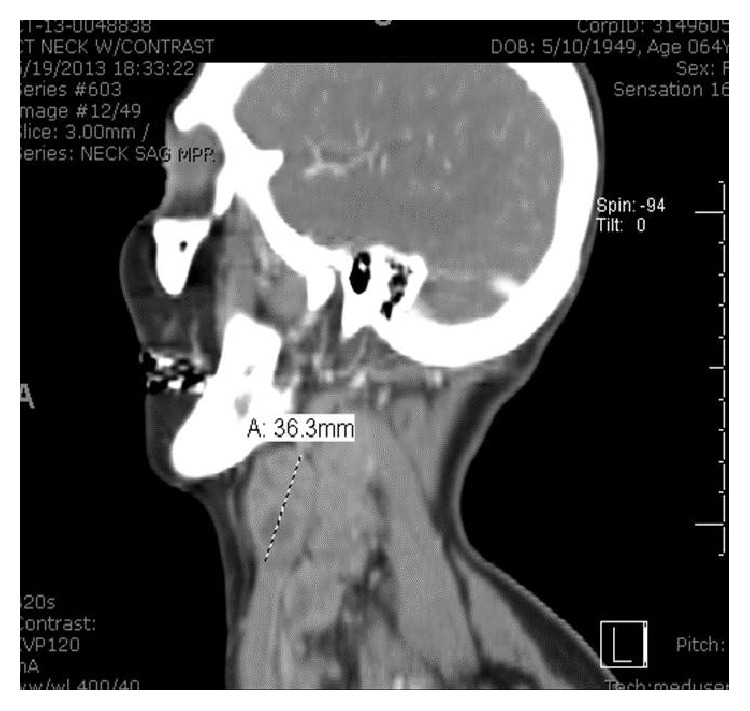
CT of the neck indicating the largest lymph node on left measuring 36.3 mm.
